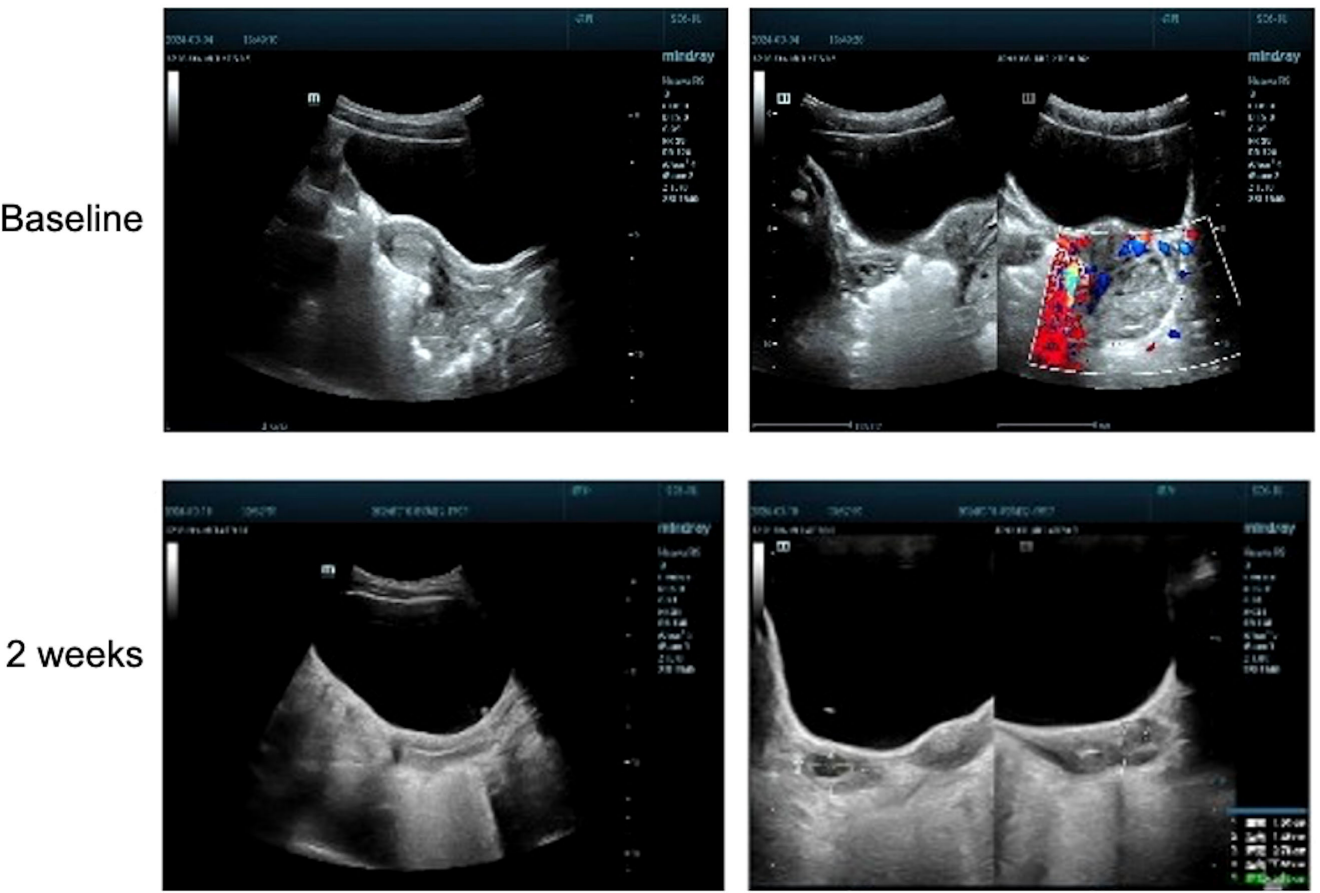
Gynecological ultrasound images acquired before (baseline) and after (2 weeks) administration of efgartigimod.
